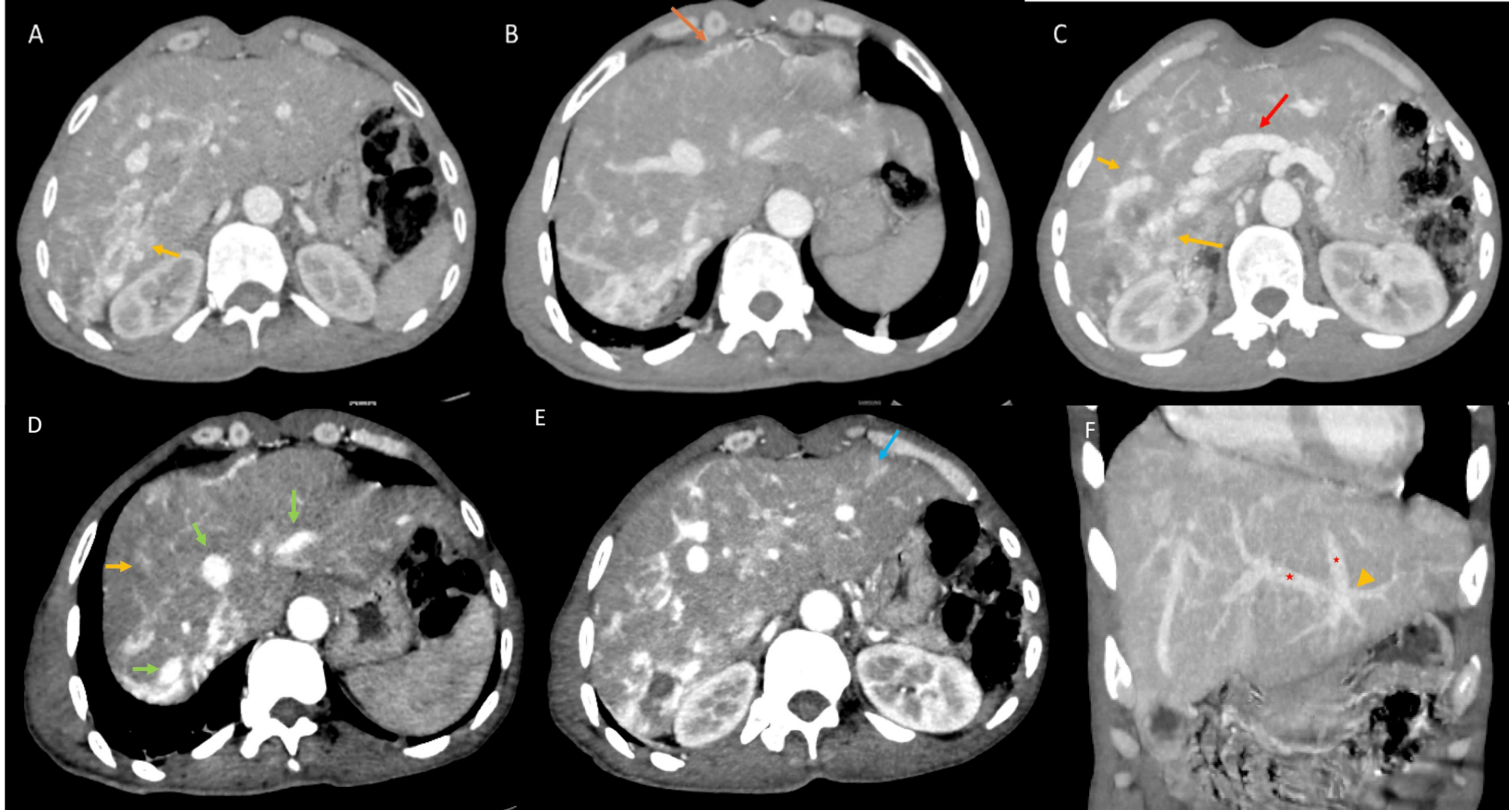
Triphasic hepatic CT-scan in axial (A,B,C,D,E) and coronal views (F) showing signs of liver involvement in hereditary hemorrhagic telangiectasia (A) Arterial phase scan showing a supra-centimetric, round, hypervascular lesion suggestive of telangiectasia (yellow arrow); (B, E) Arterial phase scan showing an early portal vein enhancement, suggesting an arterio-portal shunt, which appears as wedge-shaped areas (orange and blue arrows, respectively); (C) Arterial phase scan showing enlargement of the hepatic artery (red arrow); (D) Arterial phase scan showing early enhancement of the hepatic vein (green arrows) in the arterial phase, indicating the presence of arterio-venous shunts; (F) Portal phase scan showing communications between portal venous branches and hepatic veins (yellow arrowhead) suggest porto-venous shunts.
